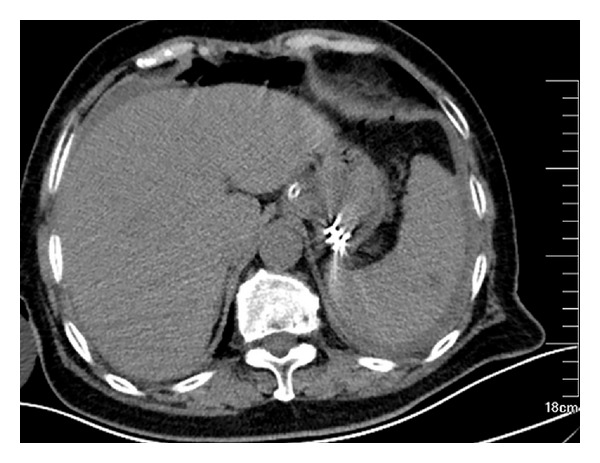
Emergent CT scanning showed a small perforation of the ligation site after the band exfoliation.
